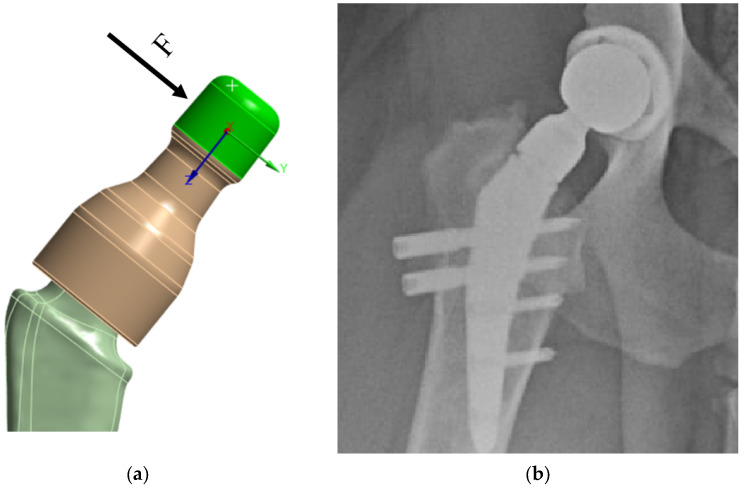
V@rt implants FEM boundary conditions: (a) load on the neck; (b) X-ray image.
